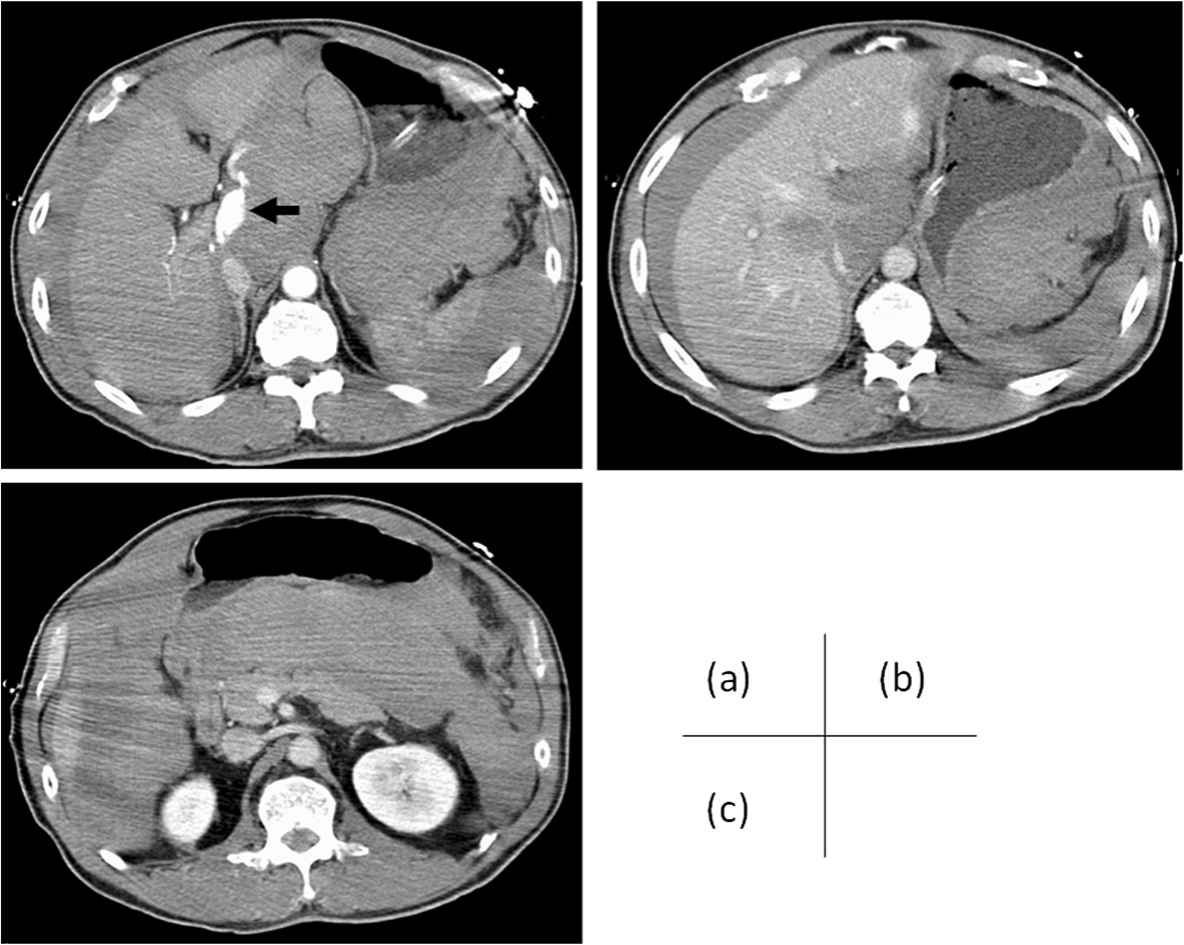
A 71-year-old man was admitted to emergency unit for abdominal trauma due to traffic accident. (a) CT showed that contrast material extravasation was in the hepatic hilum on arterial phase (arrow), and (b) an uncovered laceration extended over segments 1, 4 and 8 of the liver with massive hemoperitoneum. (c) CT scan at level at which left renal vein crosses aorta shows hemopritoneum. The ratio of anteroposterior-to-transverse diameter was equal to 1:0.76.
